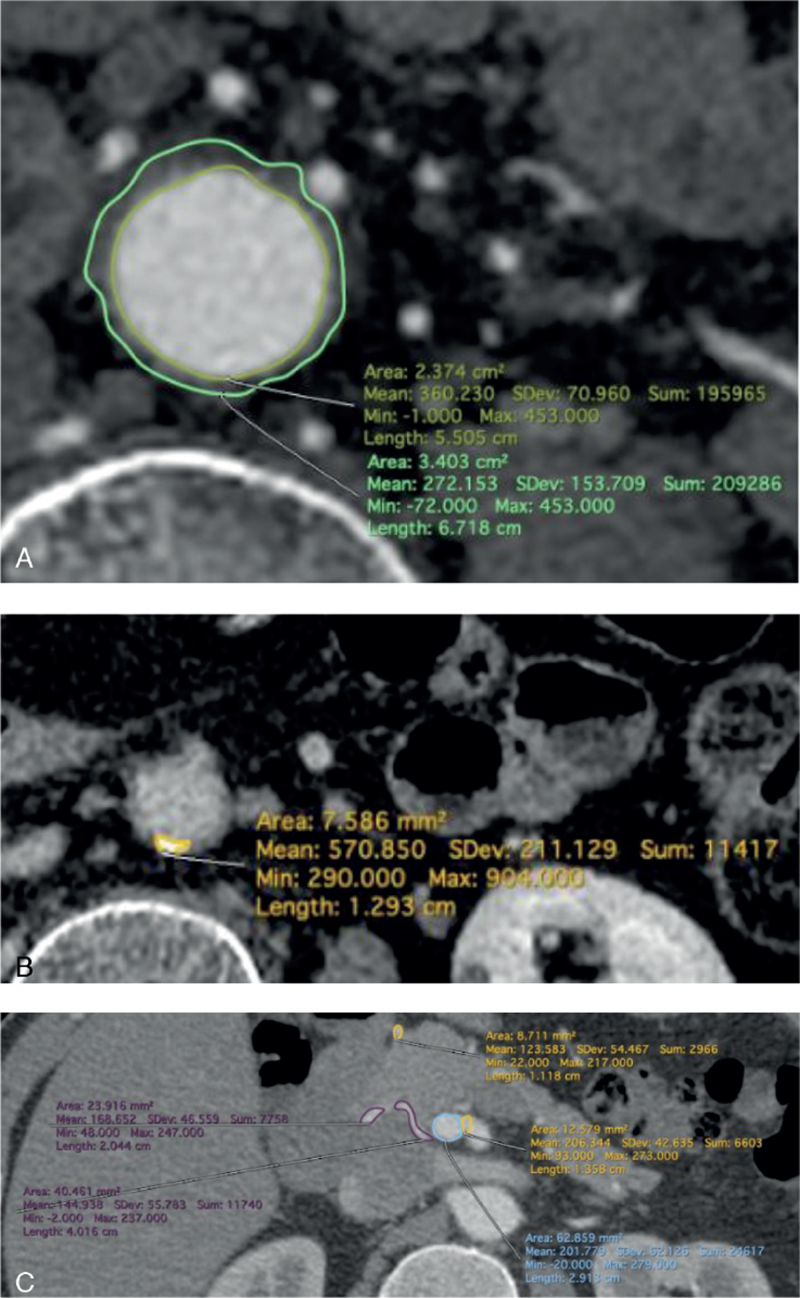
Volume calculation of CT images using Horos 3.3.5. (A) Volume of the aortic wall. (B) Volume of calcification. (C) Volume of pancreatic arcade vessels.
